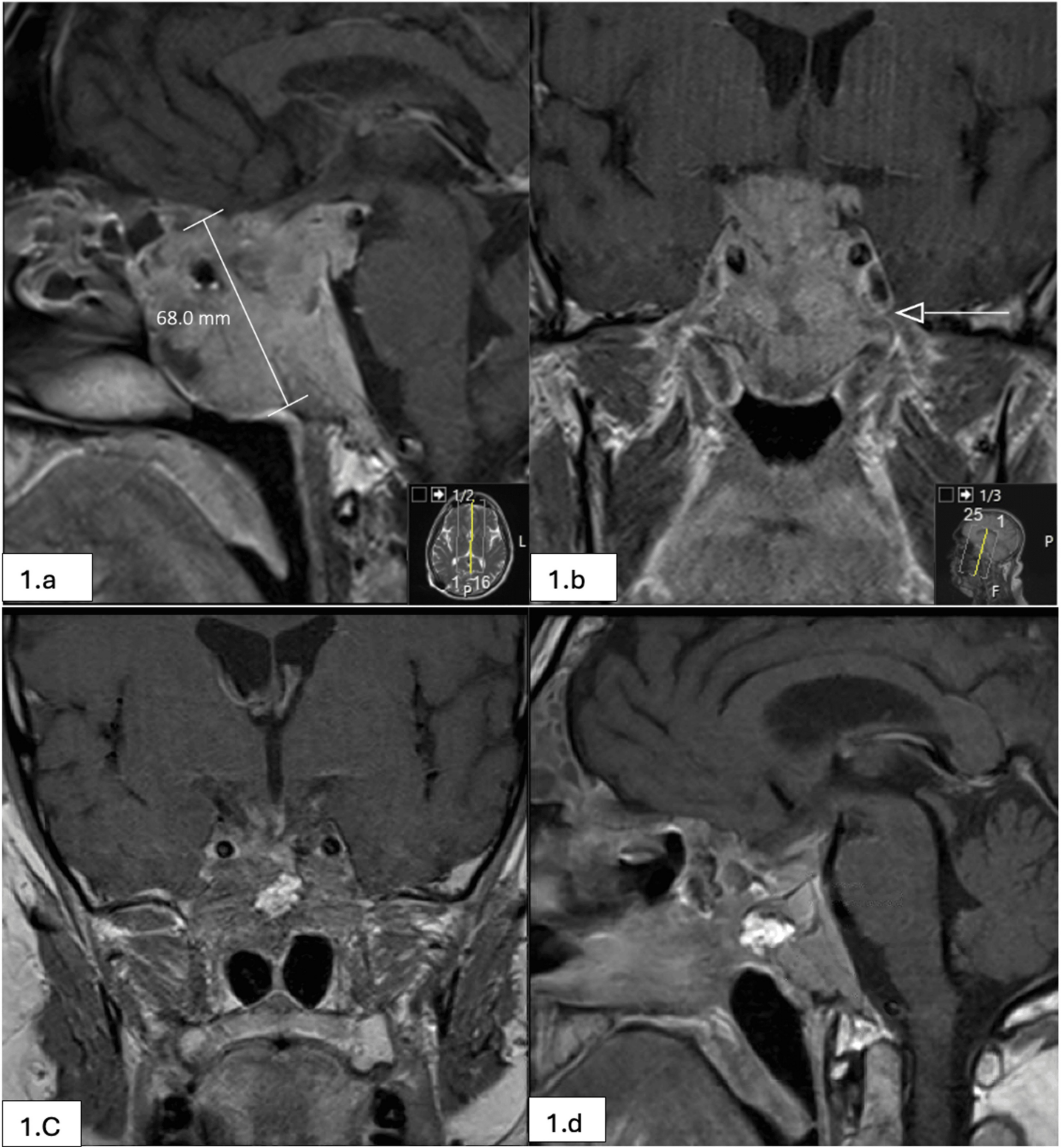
Giant pituitary adenoma with ACTH expression (pre and post-op MRI scans) (a,b): Post-contrast T1 sagittal and coronal images (1.5 Tesla MRI scanner, Siemens Healthcare Laboratory Diagnostics). A large mass lesion occupying the pituitary fossa and chiasmatic cistern and extending inferiorly to replace the sphenoid sinus. The tumor extends into the posterior ethmoid air cells, cavernous sinuses, and into the nasopharynx. Maximum diameter is 68 mm. (c,d): Post-operative MRI scan showing post-contrast T1 sagittal and coronal images (1.5 Tesla MRI scanner). ACTH, adrenocorticotropic hormone
